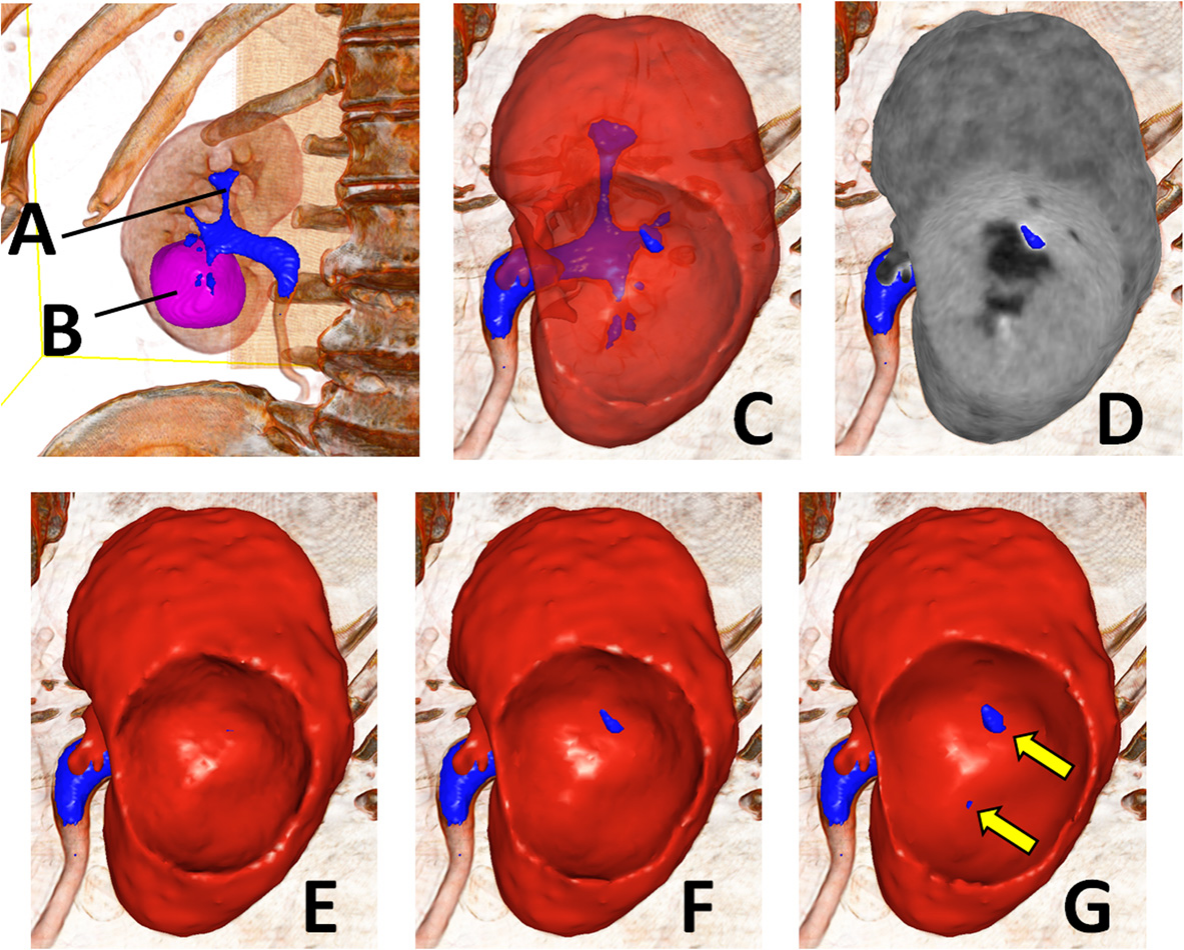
Virtual resection plane. We generated surface models of the urinary tract (A) and tumor (B) regions. We also generated multiple kidney regions that had 1 mm (E), 3 mm (F), and 5 mm (G) margins from the tumor. We also generated transparent (C) and CT-image renderings (D) of the kidney model. The CT-image rendering (D) allowed observance of the CT value on the resection plane.
